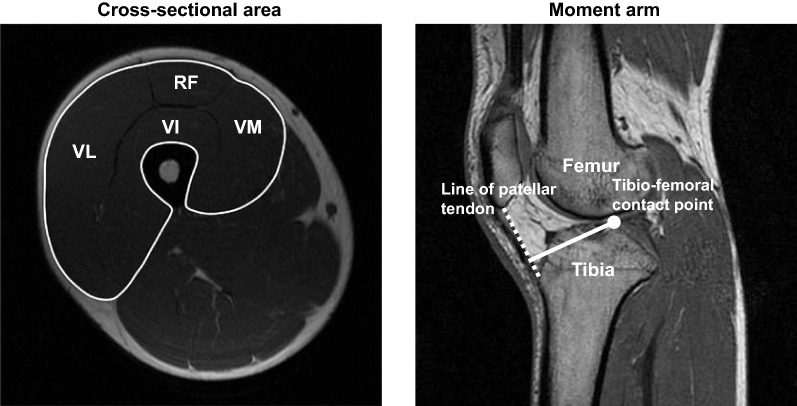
Representative magnetic resonance imaging scans of mid-thigh quadriceps femoris cross-sectional area and knee extensor moment arm. The quadriceps femoris cross-sectional area included the rectus femoris (RF), vastus intermedius (VI), vastus lateralis (VL), and vastus medialis (VM). The quadriceps femoris muscle volume was calculated by multiplying the sum of the cross-sectional areas along their length at intervals of 10 mm. The knee extensor moment arm was calculated as the distance between the tibio-femoral contact point and the mid-line of the patellar tendon
